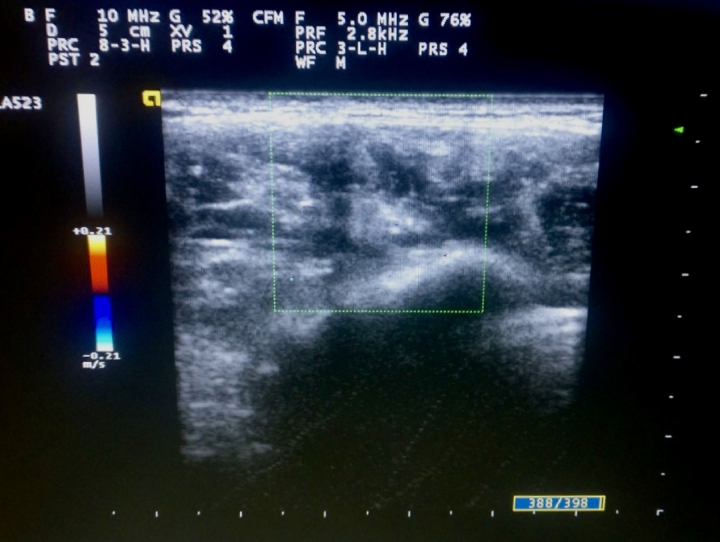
Ultrasonography showing combined vascular malformation with predominant venous malformation along with some arterial and lymphatic components
